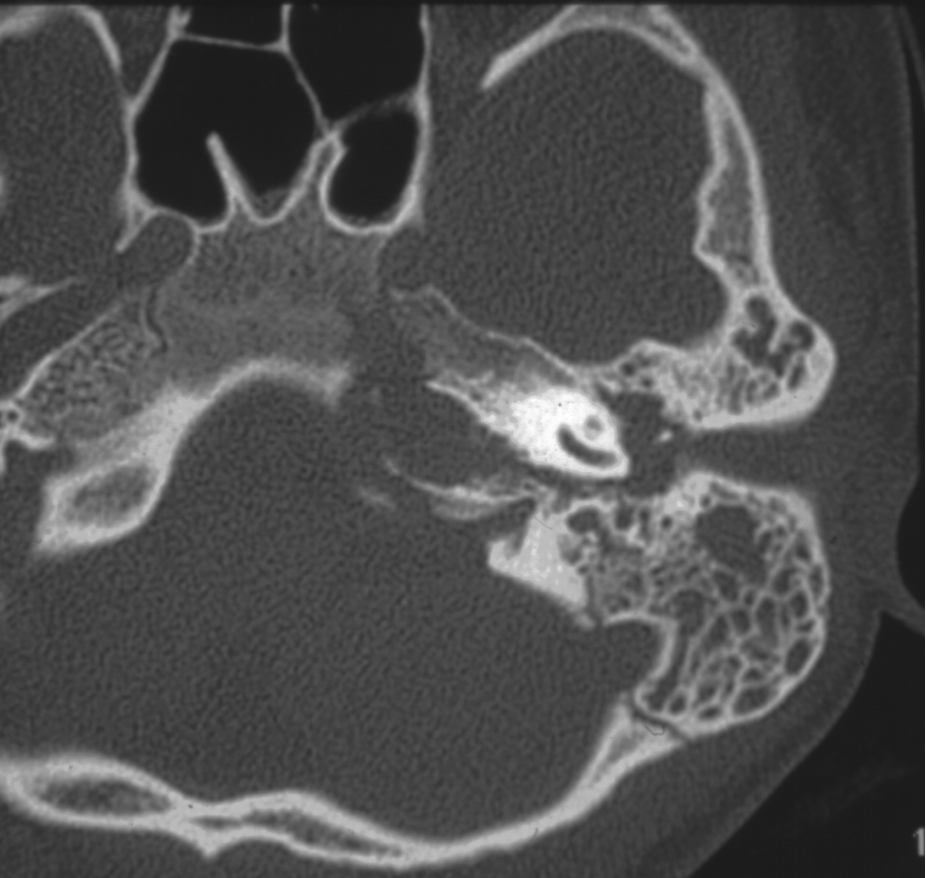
Axial computed tomography scan demonstrating a fluid-filled mastoid cavity and a hole filled with soft tissue medially to the cochlea. Notice the bone erosion of its walls.
